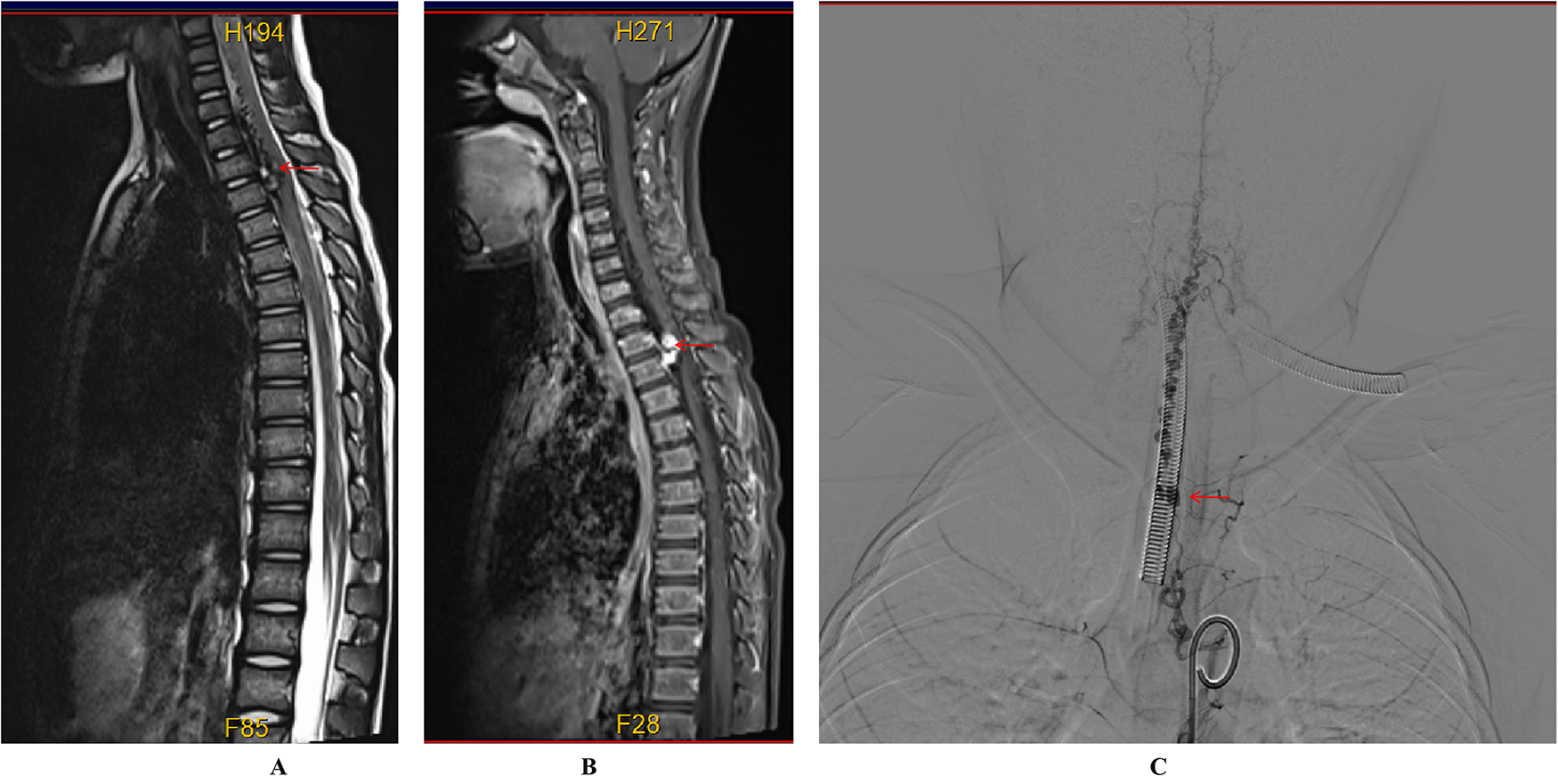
Preoperative imaging of the spinal cord vascular malformation. (A) T2-weighted MRI shows a slightly hypointense, tortuous signal beneath the extradural dura mater extending from the C4 to T3 levels. (B) Enhanced MRI reveals nodular enhancement, particularly at the T2–T3 level. (C) Digital subtraction angiography (DSA) demonstrates that the right intercostal artery at the level of the 6th to 7th rib drains into the intramedullary vein at the T2 level, with multiple tortuous and thickened vascular networks extending from the cervical segment to the T2 level of the spinal cord.
